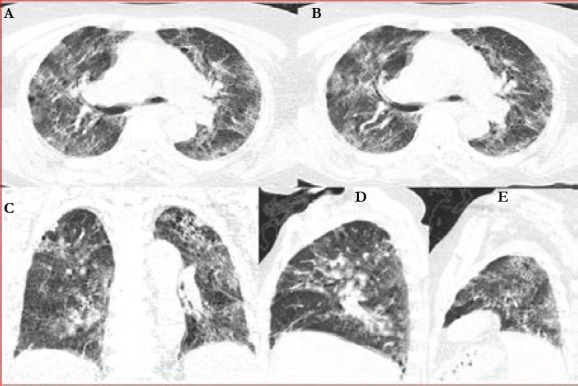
Axial (A, B), coronal(C), and sagittal (D, E) chest HRCT-lung windows of a 51-year-old male showing ground glass opacities with interlobular septal thickening and crazy paving pattern. This patient was categorised as CORADS 5, stage 3 with a severity score of 21/25
